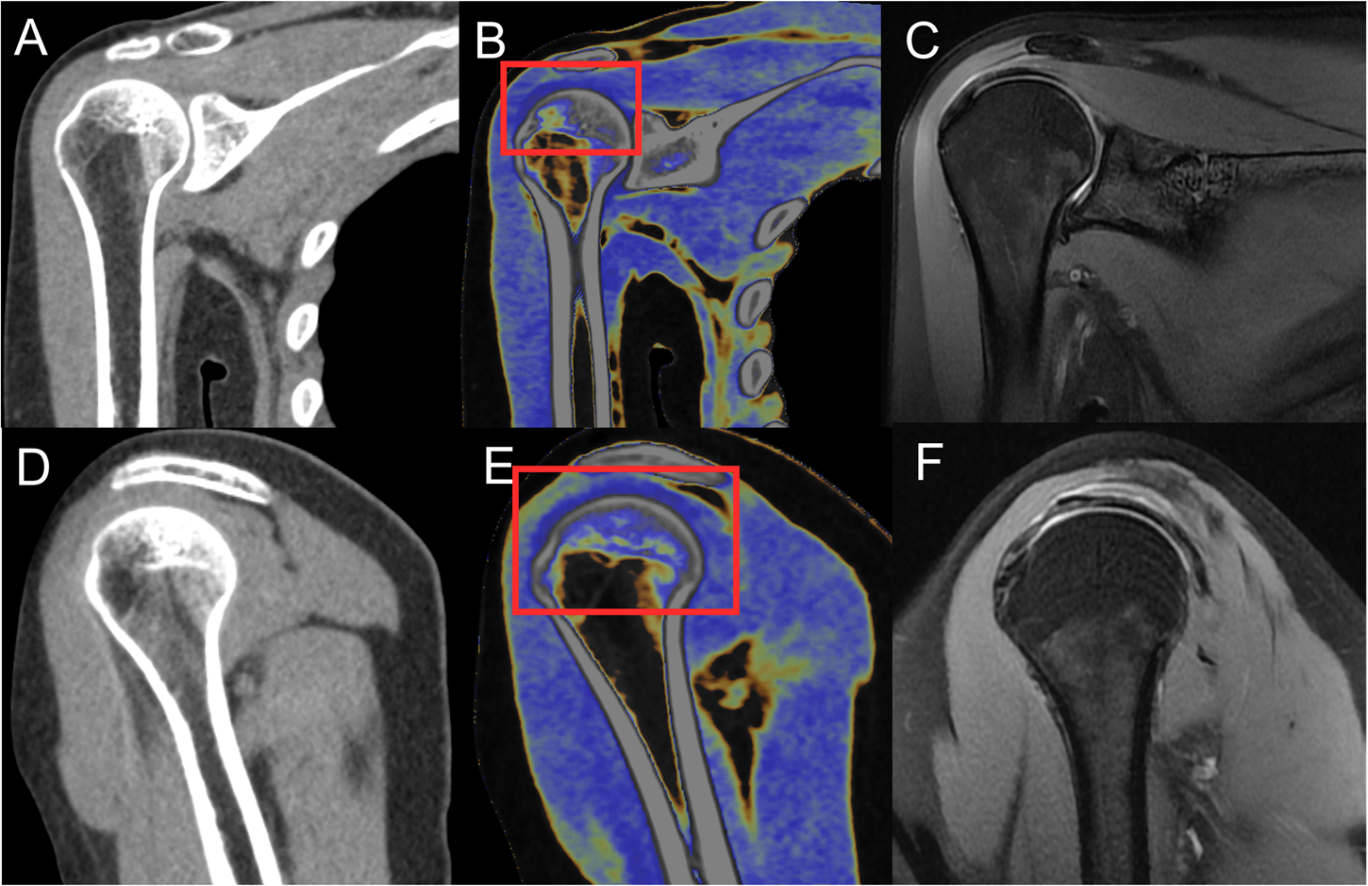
Images for a 26-year-old woman with a normal shoulder. A, D At standard CT, the rotator cuff is indistinct. B, E After MMD reconstruction images, the normal rotator cuff is dark blue (red rectangular). C, F Noncontrast MRI in oblique coronal and oblique sagittal positions demonstrates a normal rotator cuff on fat-suppressed proton density-weighted imaging. MMD, multi-material decomposition
